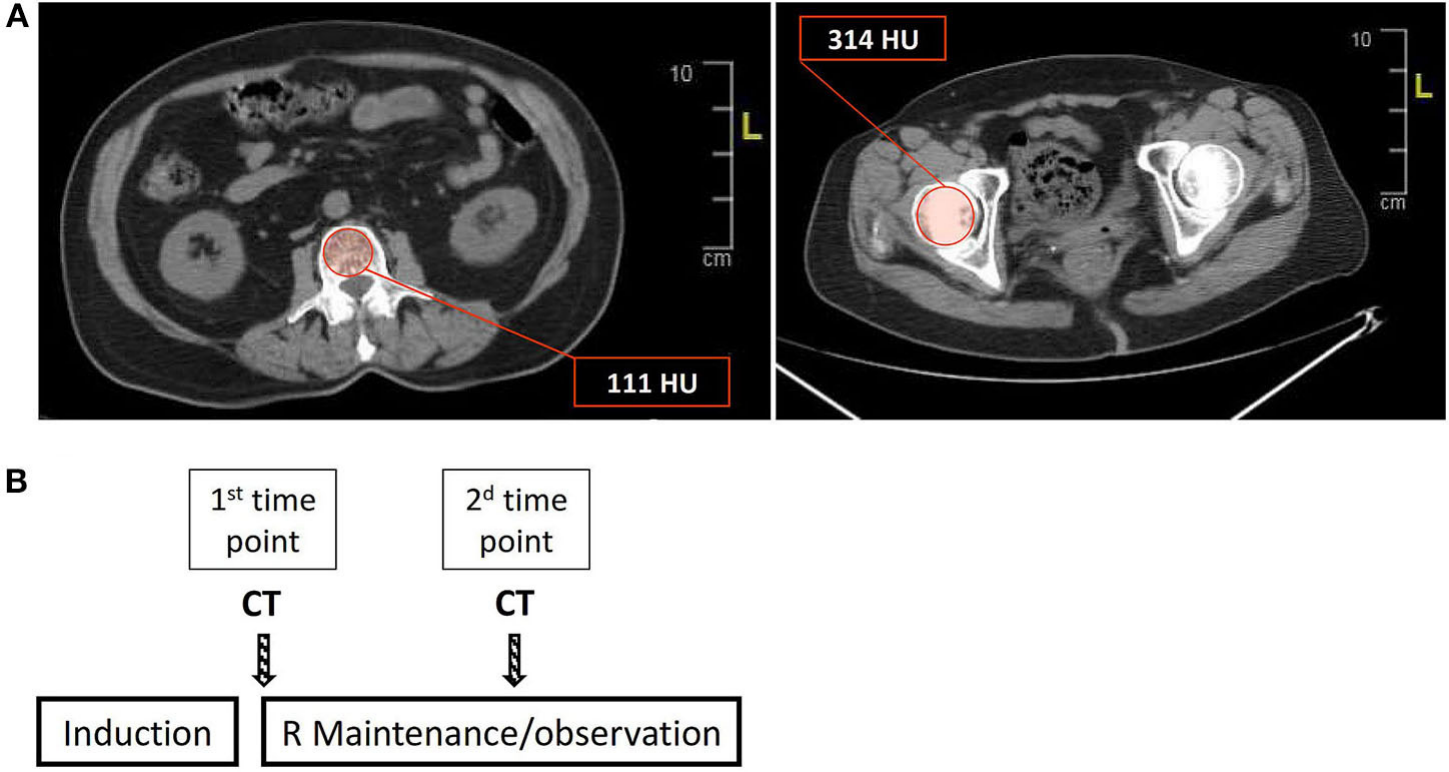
Estimation of bone mass using cross-sectional CT-based imaging. (A) Bone mineral density (BMD) was measured in the lumbar spine (L1–L4) and femoral head, using regions of interest (ROIs) as depicted (red circle) in the left and right panels for the lumbar vertebrae (L1 in this section) and femoral head, respectively. The values in the red boxes reflect the average of the HU (Hounsfield units) values in the ROI (see also “Methods” for a detailed description of BMD calculation). (B) A scheme describing the timing of the sequential BMD measurements, that is, the timing of the CT/PET scans in relation to the treatment steps. R = rituximab.
